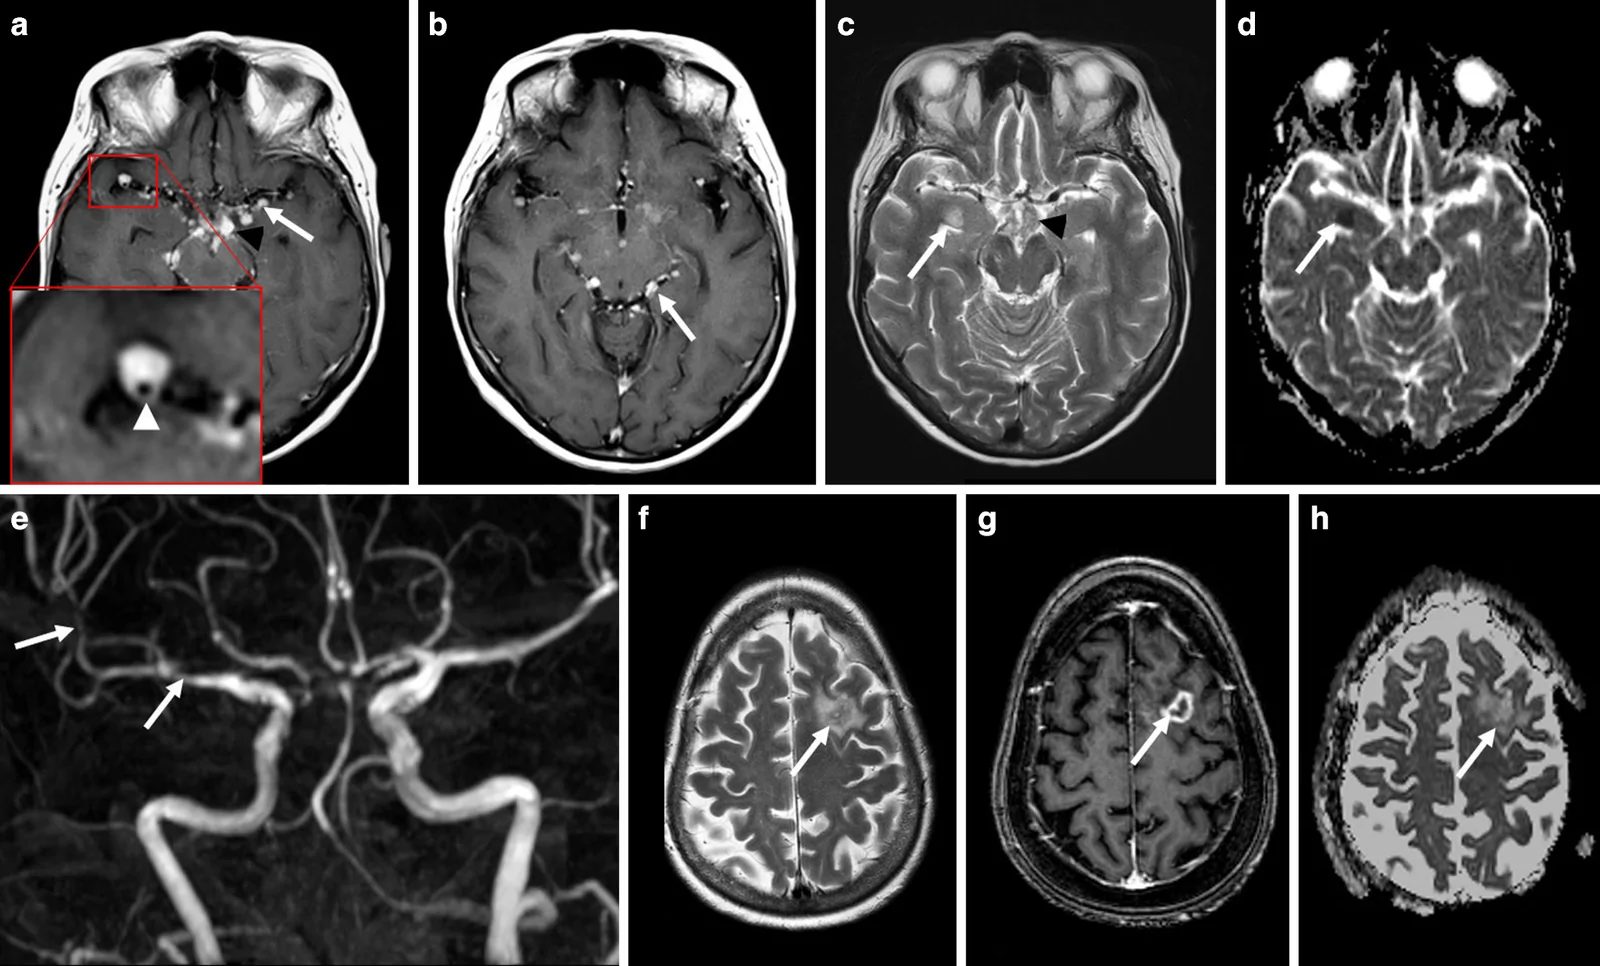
CNS tuberculosis. a–e multiple nodular enhancing lesions especially in the basal cisterns (a, b arrow; post contrast T1 weighted images (WI)), partially encasing basal arteries (a box: arrowhead) and cranial nerves (a, c arrowhead; oculomotor nerve); small temporal infarct (c, d arrow; c T2 WI; d DWI/apparent diffusion coefficient (ADC) map; ADC-value: 0.523 ± 0.038 × 10^−3^ mm^2^/s; mean ± standard deviation (SD)) and several vascular stenoses on MR angiography (e arrow); f–h intraaxial tuberculous granuloma (arrow) frontodorsal left with inhomogeneous central T2 hyperintense (f) und T1 hypointense (g) signal changes, marginal contrast enhancement (g arrow) and partially increased ADC-value (1.44 ± 0.12 × 10^−3^ mm^2^/s; mean ± SD; h arrow) indicating caseating tuberculoma with liquefaction
